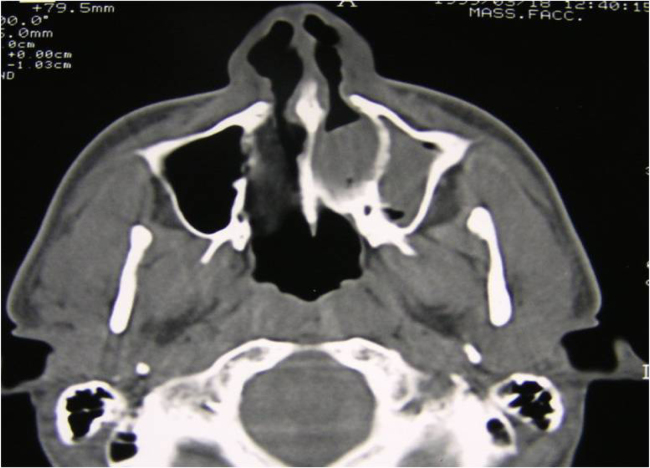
Axial CT scan, monolateral left bony choanal atresia.
